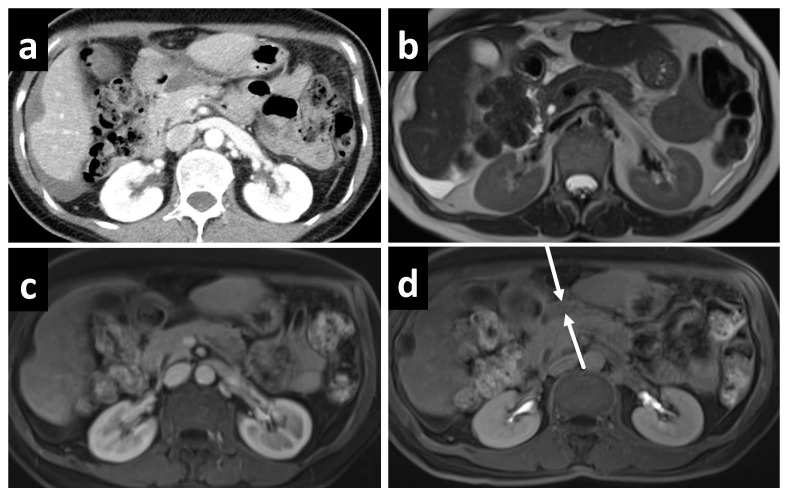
A 61-year-old female with mucinous appendiceal neoplasm, a small amount of fluid is noted within the lesser sac on (a) CT and (b) T2W MRI. Enhancement within the fluid, however, is barely appreciated on the (c) portal venous phase of the MRI, but becomes clearly apparent on the (d) delayed MRI.
